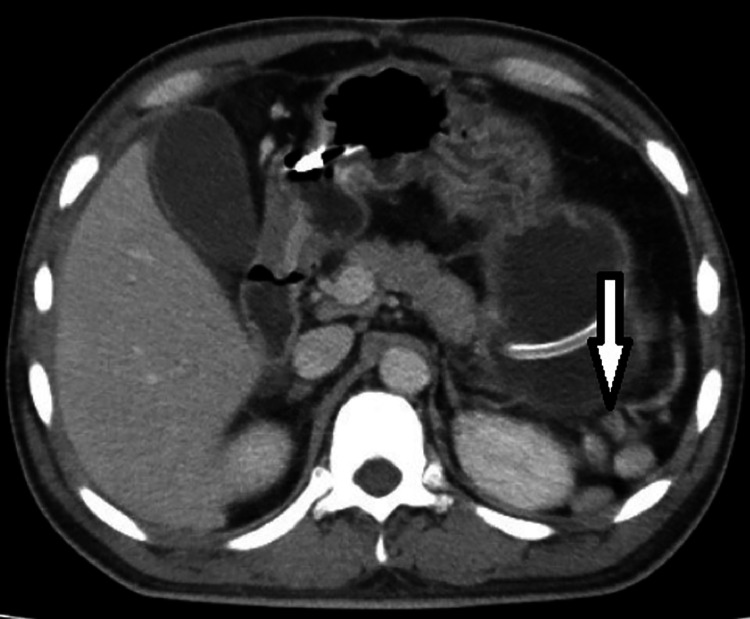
Axial contrast enhanced CT image showing splenic implants in left lumbar region.
